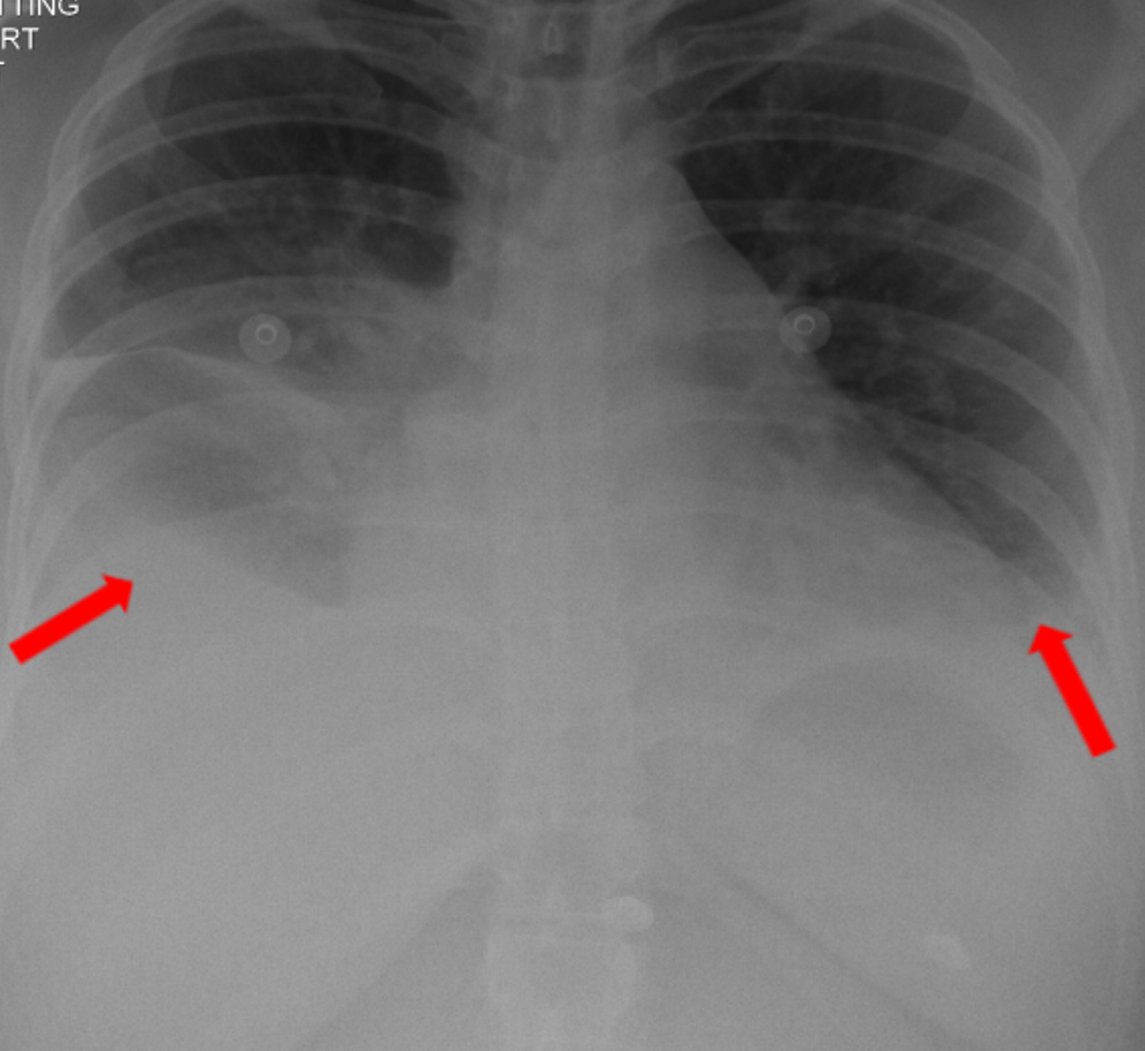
Chest radiograph AP view showing bilateral pleural effusion (Red arrows), with underlying collapse consolidation.
